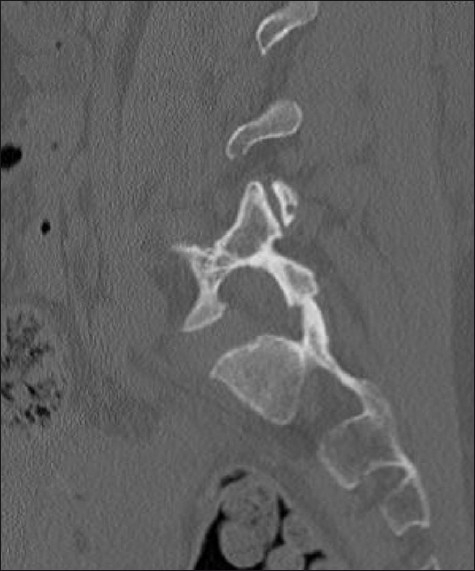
Computed tomography of the lumbar spine. Sagittal reconstruction through the perched right L5–S1 facet joint
